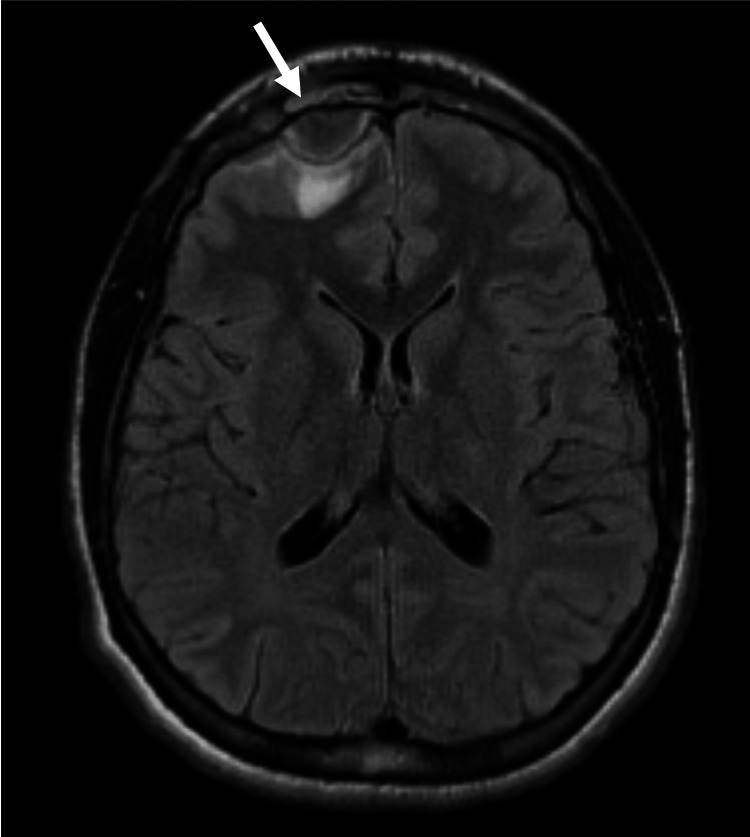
MRI showed 2 cm intracranial abscess at the right frontal convexity with mass effect on the adjacent right frontal lobe and adjacent cerebral edema and potentially cerebritis.
